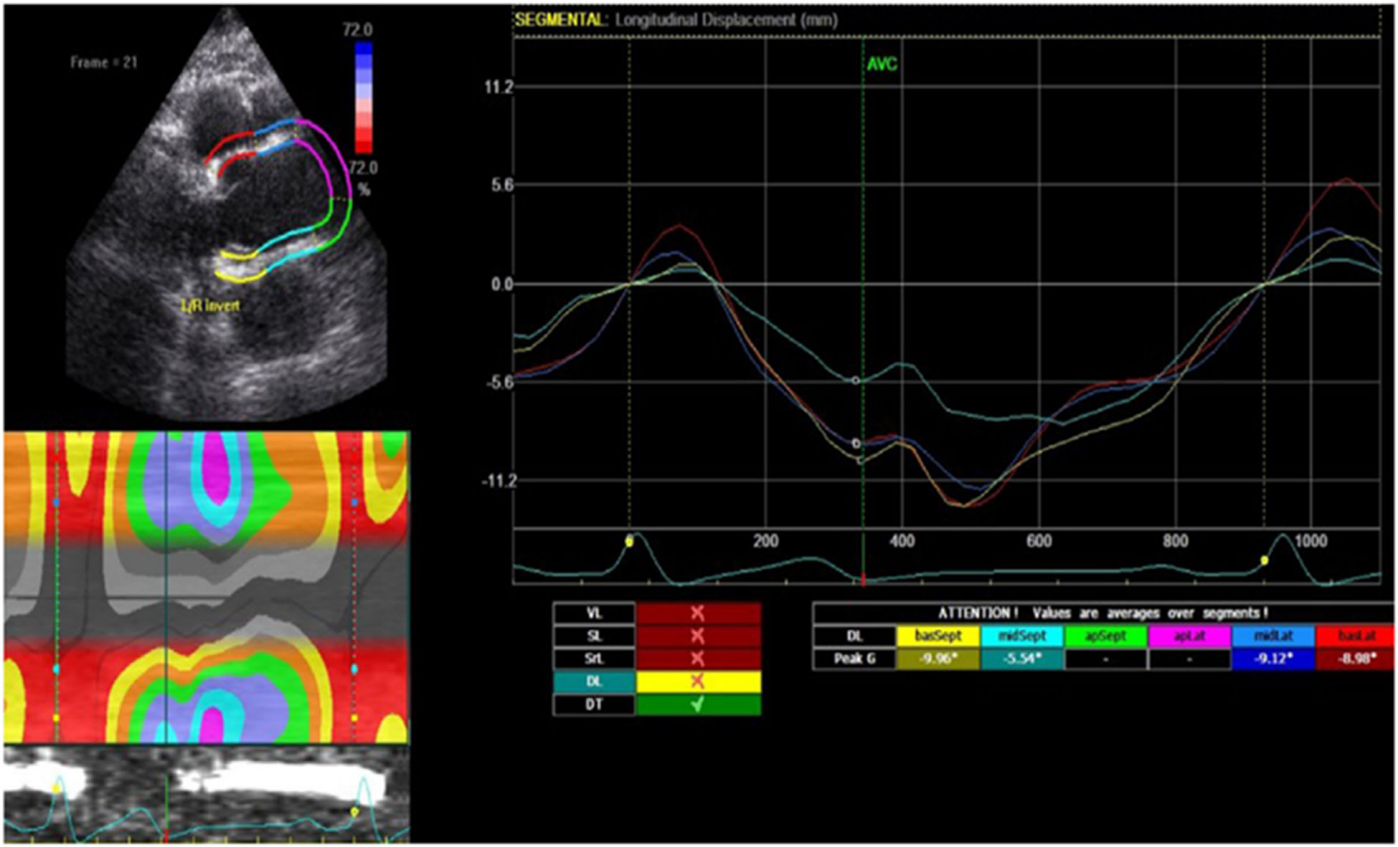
Example parasternal long axis view of a two-dimensional speckle-tracking echocardiographic image of longitudinal displacement of the ascending aorta
